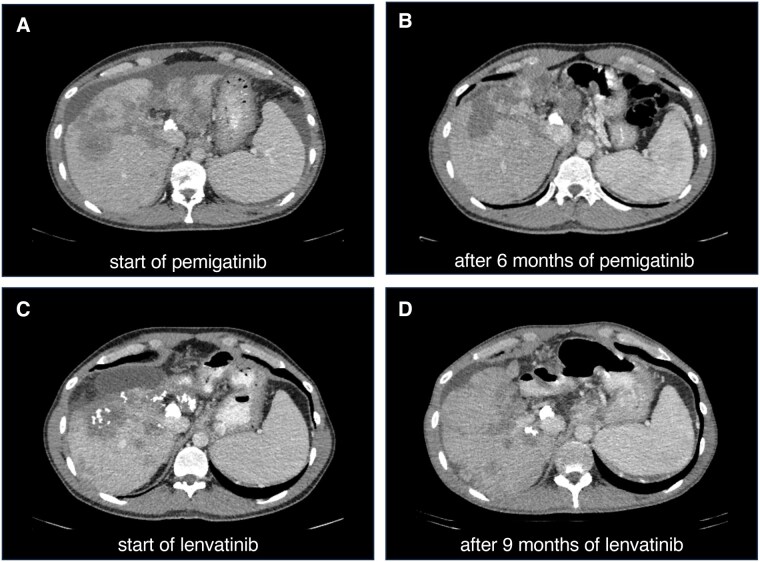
CT scans showing progressive disease under FOLFIRINOX therapy (A) and subsequent stable disease after 6 months of pemigatinib therapy (B). Following progression on pemigatinib and detection of an FGFR kinase point mutation, the tumor progressed further under futibatinib therapy (C). Disease remained stable after 9 months of lenvatinib treatment (D). Tumor response was evaluated according to RECIST 1.1 criteria.
